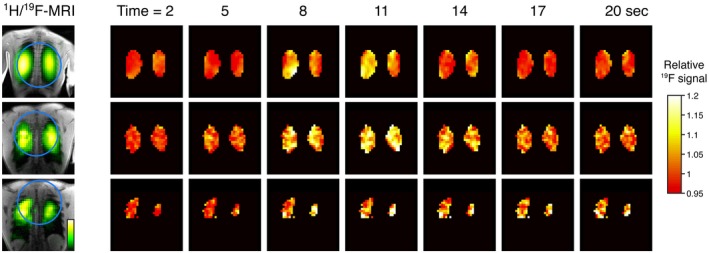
Dynamic ^19^F‐MRI of inhaled PFP acquired before, during, and after passage of a GBCA bolus through the pulmonary vasculature in 3 healthy volunteers. $T_{2}^{*}$‐weighted ^19^F‐MRI images overlaid on ^1^H anatomical scans (left) show blue circles to indicate position of the ^19^F surface coil. Dynamic ^19^F‐MRI data (right) show relative change in $T_{2}^{*}$‐weighted ^19^F‐MRI signal compared to baseline amplitude (i.e., pre‐GBCA bolus arrival). A transient elevation in PFP signal amplitude is observed at approximately 8 to 14 s following GBCA administration, reflecting partial susceptibility matching of lung water and gas components
